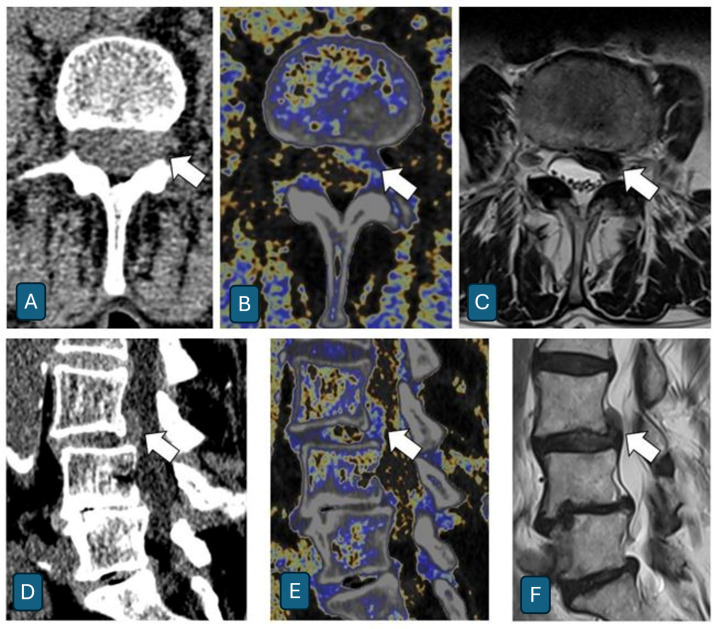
A 55-year-old male patient with a history of left-sided lumbar radiculopathy/lumbalgia. Axial and sagittal imaging demonstrates a posterolateral left-sided extruded disk component with partial cranial migration beneath the posterior longitudinal ligament (white arrow). Compared to conventional CT images (A,D), DECT images (B,E) enhance the contrast differentiation between discal structures (in blue) and cerebrospinal fluid (in grayscale), allowing an optimal diagnostic assessment. MRI T2 images (C,F) represent the current gold standard.
